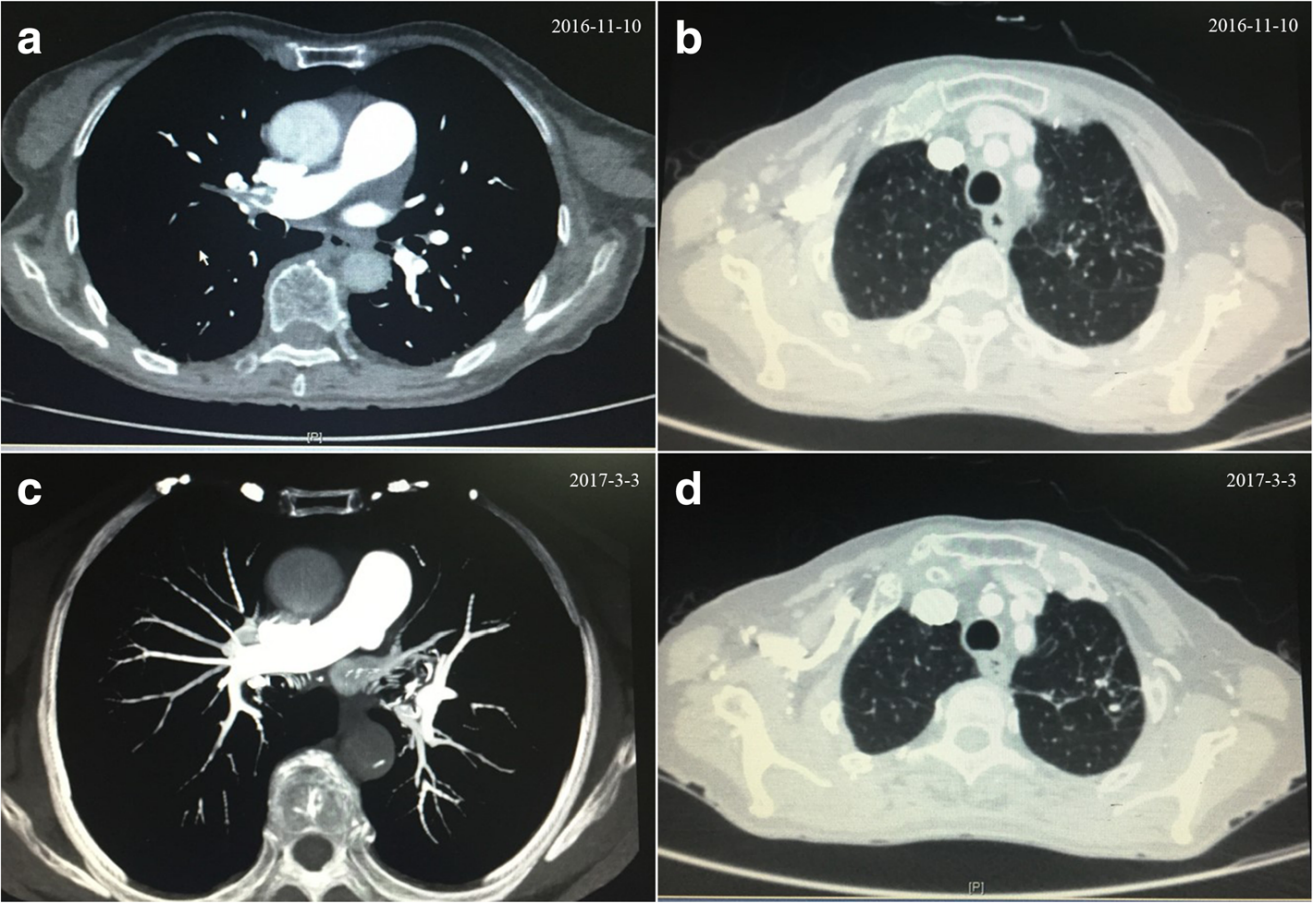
Comparison of chest CT on Day 3 after admission (a and b) and at 3 months follow-up (c and d)
